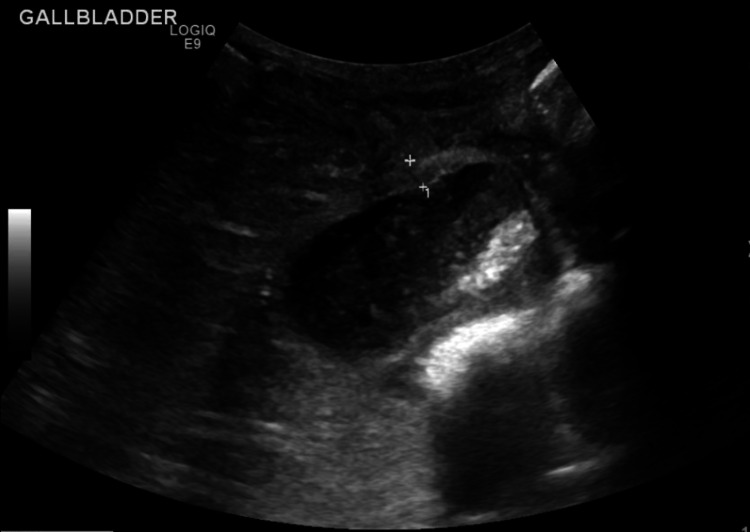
Ultrasound showing biliary sludge and some peri-cholecystic fluid.
